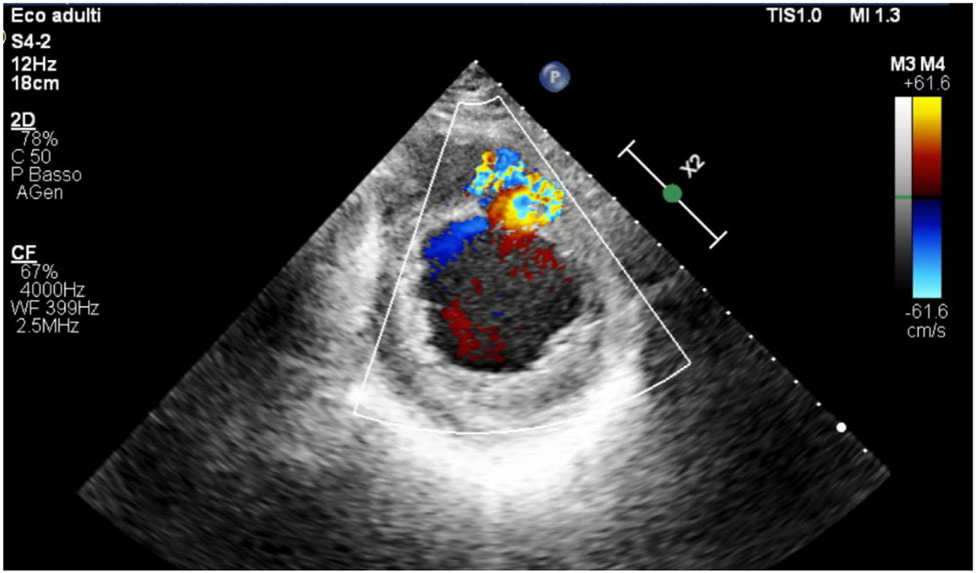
Transthoracic Doppler US showing blood flow across the ventricular septal defect
